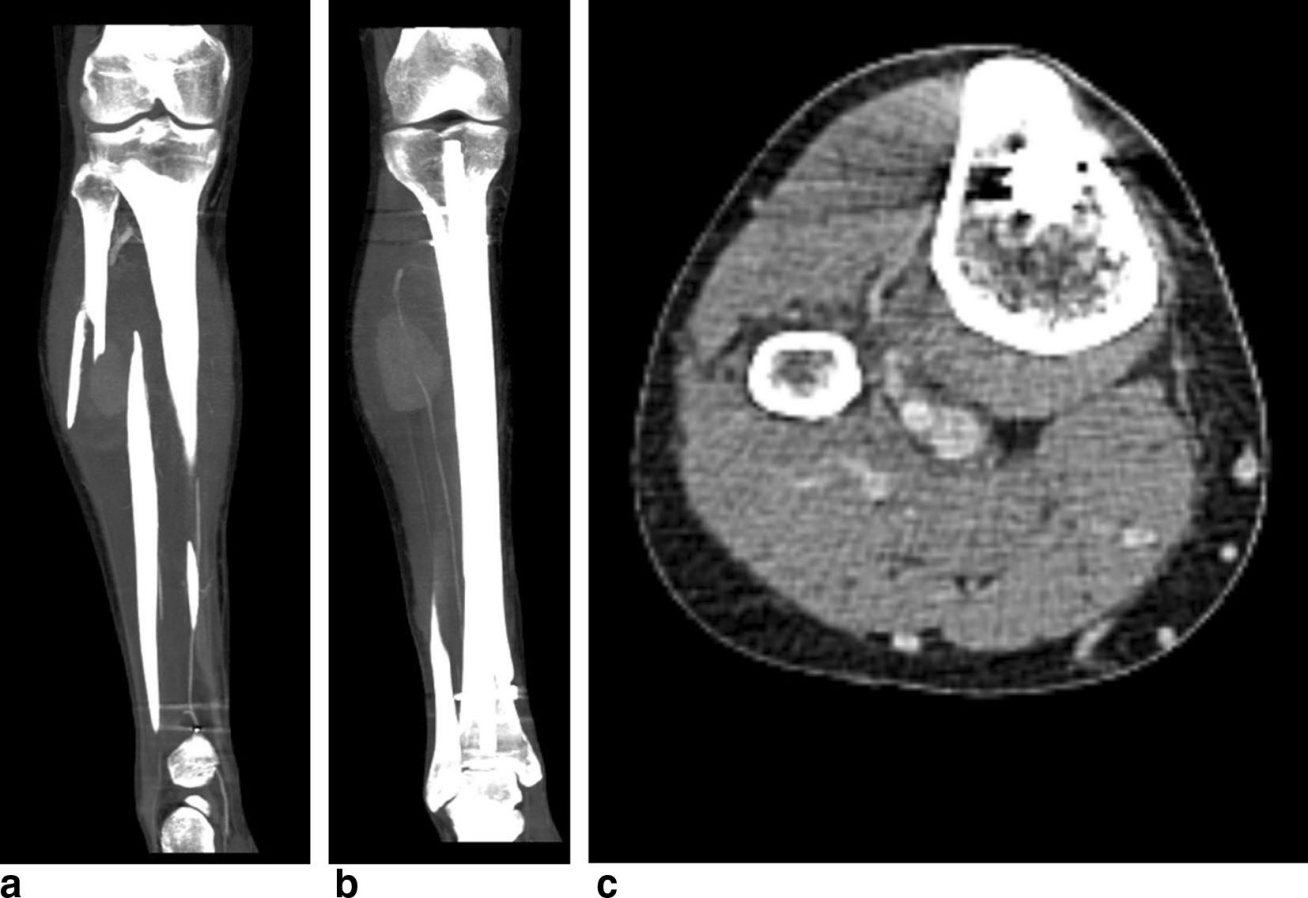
CT angiography of the lower leg showing the pseudoaneurysm (a) at the level of proximal screw insertion (b, c)
